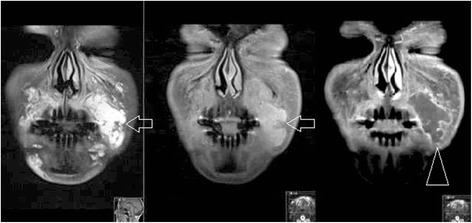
Abscess formation in the left nasolabial sulcus region (*open arrows*) following injection of polyacrylamide, coronal MRI (T2FS, T1FS and T1FS after injection of gadolinium). Note the peripheral “ring-like” enhancement after administration of gadolinium, typical of an abscess (*arrowhead*)
